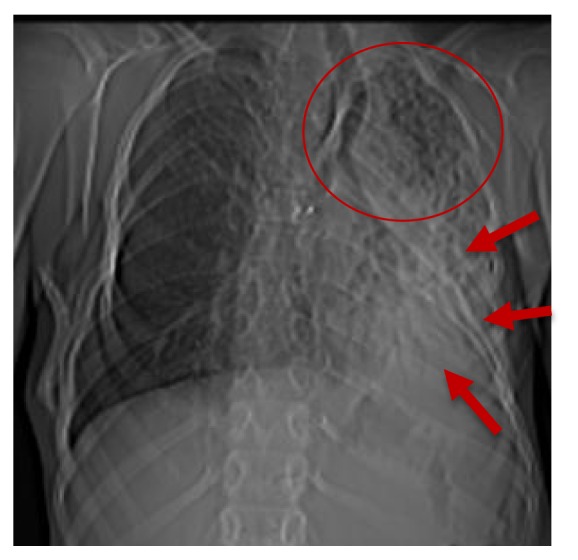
Frontal chest x-ray showing an atelectasis of the left lower lobe (red circle) with alvéolo-interstitial pneumopathy on the homolateral upper lobe (red arrows).
